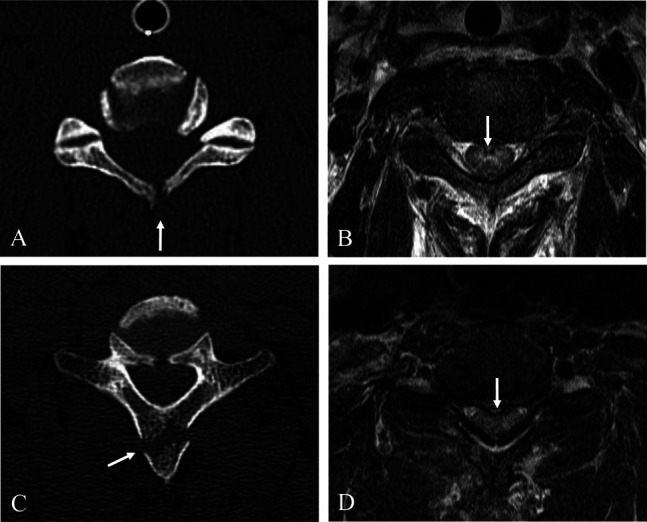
Axial CT scan (A) and magnetic resonance imaging (B) at C3-4 showing lamina fracture of C4 (white arrow) and intramedullary hemorrhage of C4 spinal cord (white arrow). Axial CT scan (C) and magnetic resonance imaging (D) at C6-7 show spinous process fracture of C7 (white arrow) and intramedullary hemorrhage of C7 spinal cord (white arrow).
